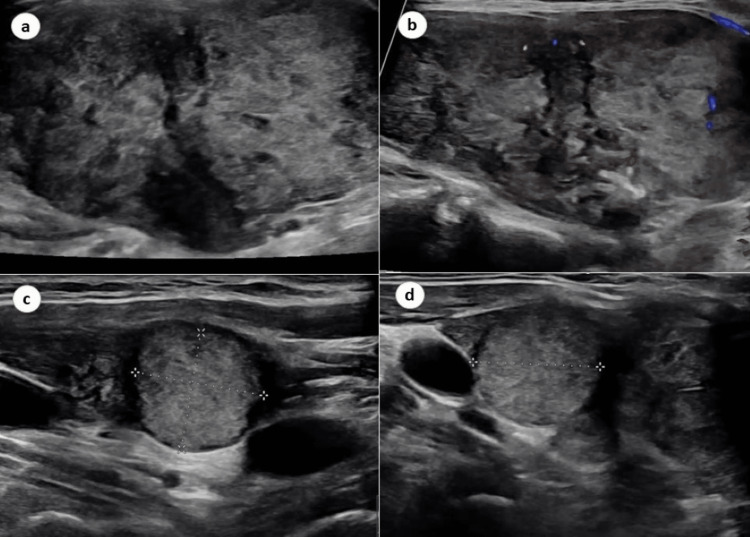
Ultrasound of the thyroid gland demonstrating (a, b) right lobe (5.7 x 2.8 x 4.4 cm) and the left lobe (1.4 x 0.3 x 0.6 cm), (c) a right lateral lower pole isoechoic solid and cystic nodule measuring 1.6 x 1.4 x 1.5, and (d) a right mid pole mixed echogenicity solid and cystic nodule measuring 5.3 x 2.7 x 4.3 cm.
